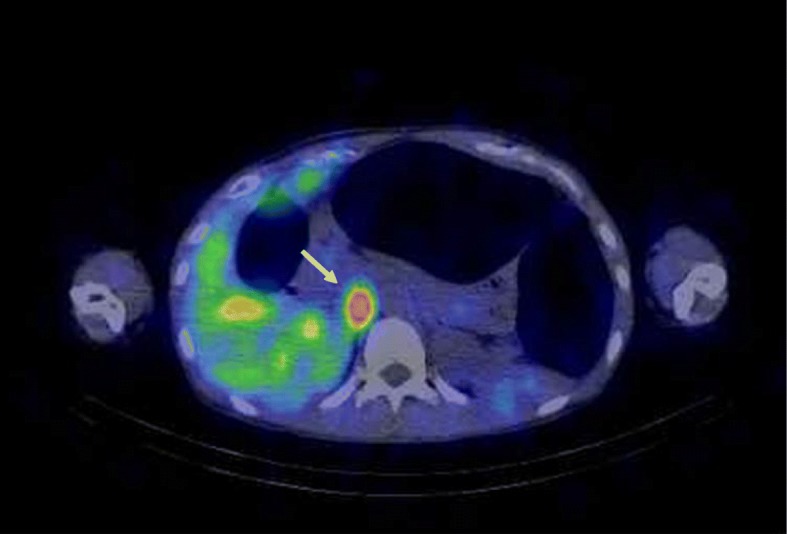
^123^I-metaiodobenzylguanidine scintigraphy shows a 17 × 15 mm mass accumulation on the left of the inferior vena cava (white arrows)
